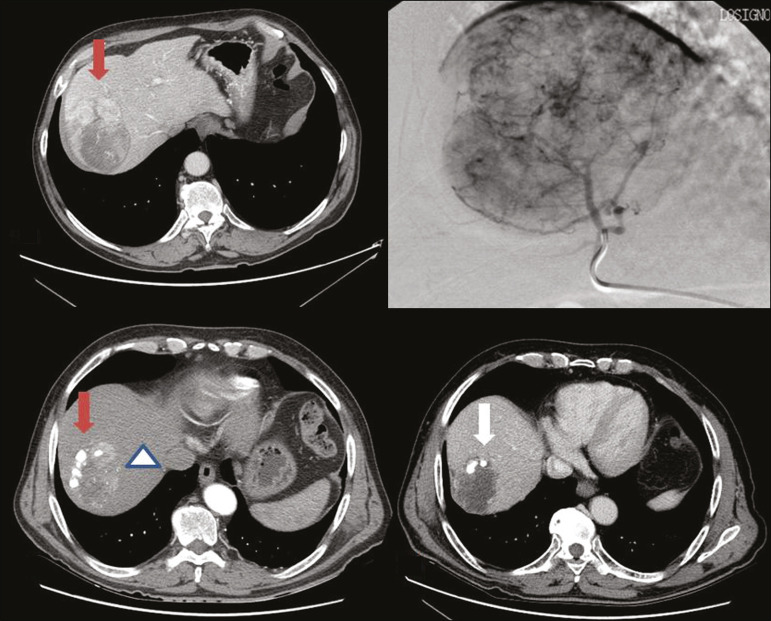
Conventional TACE of a large HCC. Contrast-enhanced computed tomography scan of a 71-year-old patient with liver cirrhosis and a large (8 cm) HCC involving the right liver lobe (red arrow). Right hepatic artery branch digital subtraction angiography showing the hypervascular lesion. Follow-up contrast-enhanced computed tomography scan, acquired one month later, demonstrating a partial response, with tumour necrosis (white arrow), lipiodol deposition, and residual tumour in the lower part of the nodule (arrowhead).
